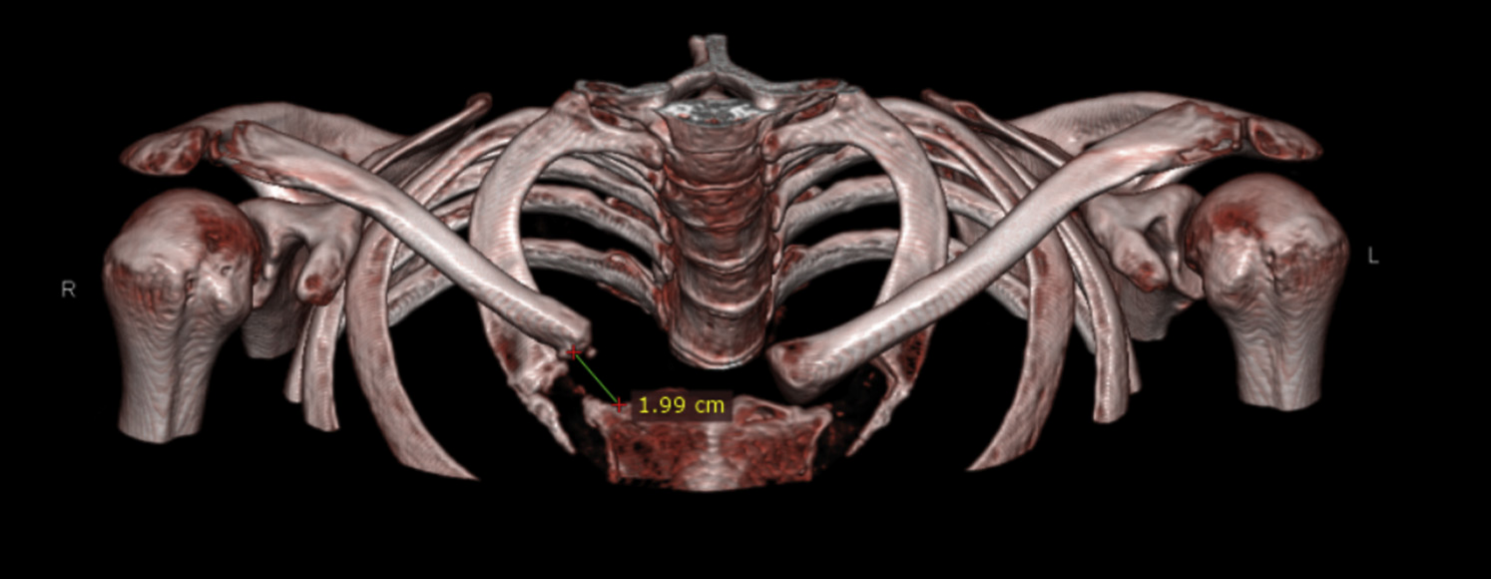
Three-dimensional reconstruction of the shoulder girdle from computed tomography. The defect after medial right clavicular resection is measured at 1.99 cm.
